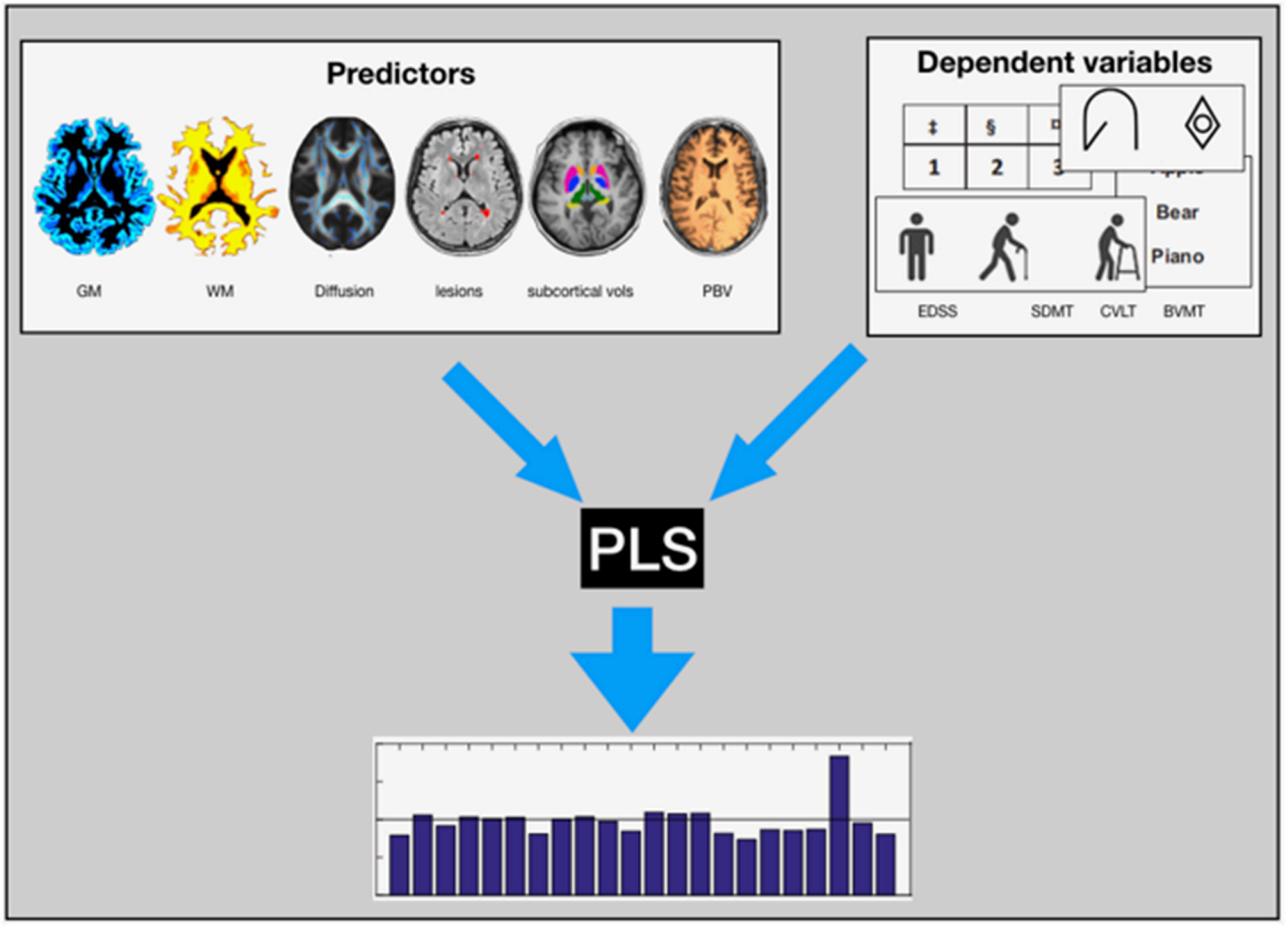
Graphical Presesntation: We used PLS regression analysis to estimate the contributions of the various MRI parameters (GM, WM, the diffusion parameters of the lesions and the normal appearing white matter, the Lesion Load, volume of the subcortical structures, and the PBV) to the EDSS and the subscores of the BICAMS test.
